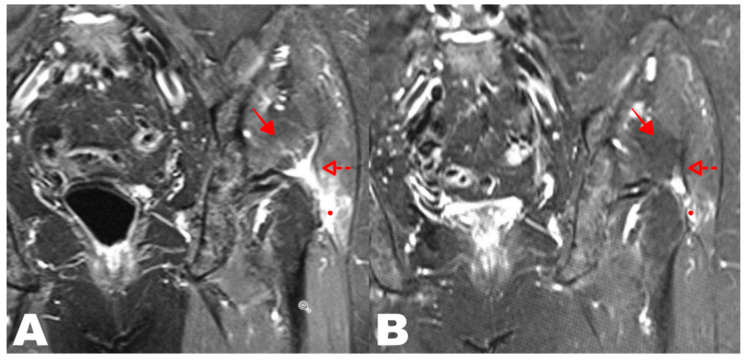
(A) Pre-treatment MRI coronal STIR sequence of a patient’s left hip affected by GTPS with the presence of a fluid signal in the insertional area of the gluteus medius tendon; (B) MRI coronal STIR sequence of the same hip at T24s/FU with an apparent reduction of fluid. The full point indicates the bursal effusion; intra-tendinous edema is indicated by the dotted arrow, and muscle edema is indicated by the full arrow.
